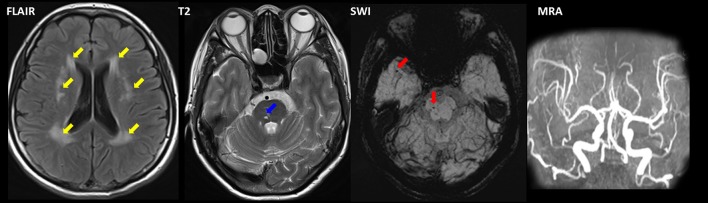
Magnetic resonance imaging of II-1 with combined dHMN-V and cerebral small vessel disease (CSVD). Fluid-attenuated inversion recovery (FLAIR) imaging revealed a hyperintensity (yellow arrow) surrounding the lateral ventricle and the bilateral centrum semiovale. Microbleeds (red arrow) and lacunar infarction (blue arrow) were present in the brainstem as observed using susceptibility weighted imaging (SWI) and T2. Magnetic resonance angiography (MRA) revealed normal intracranial arteries.
